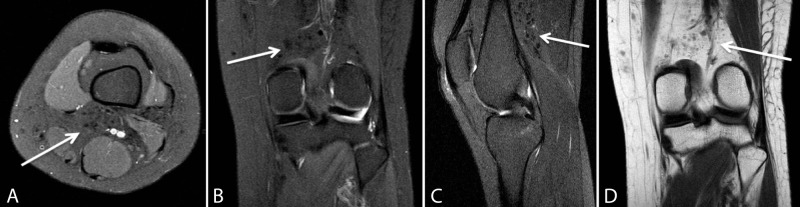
MRI Left Knee Axial proton-density *(A)*, coronal proton-density *(B)*, sagittal T2-weighted *(C)*, and coronal T1-weighted *(D)* MR images of the left knee demonstrate translocated free-silicone and reactive granuloma formation *(arrows) *within the posterior knee.
